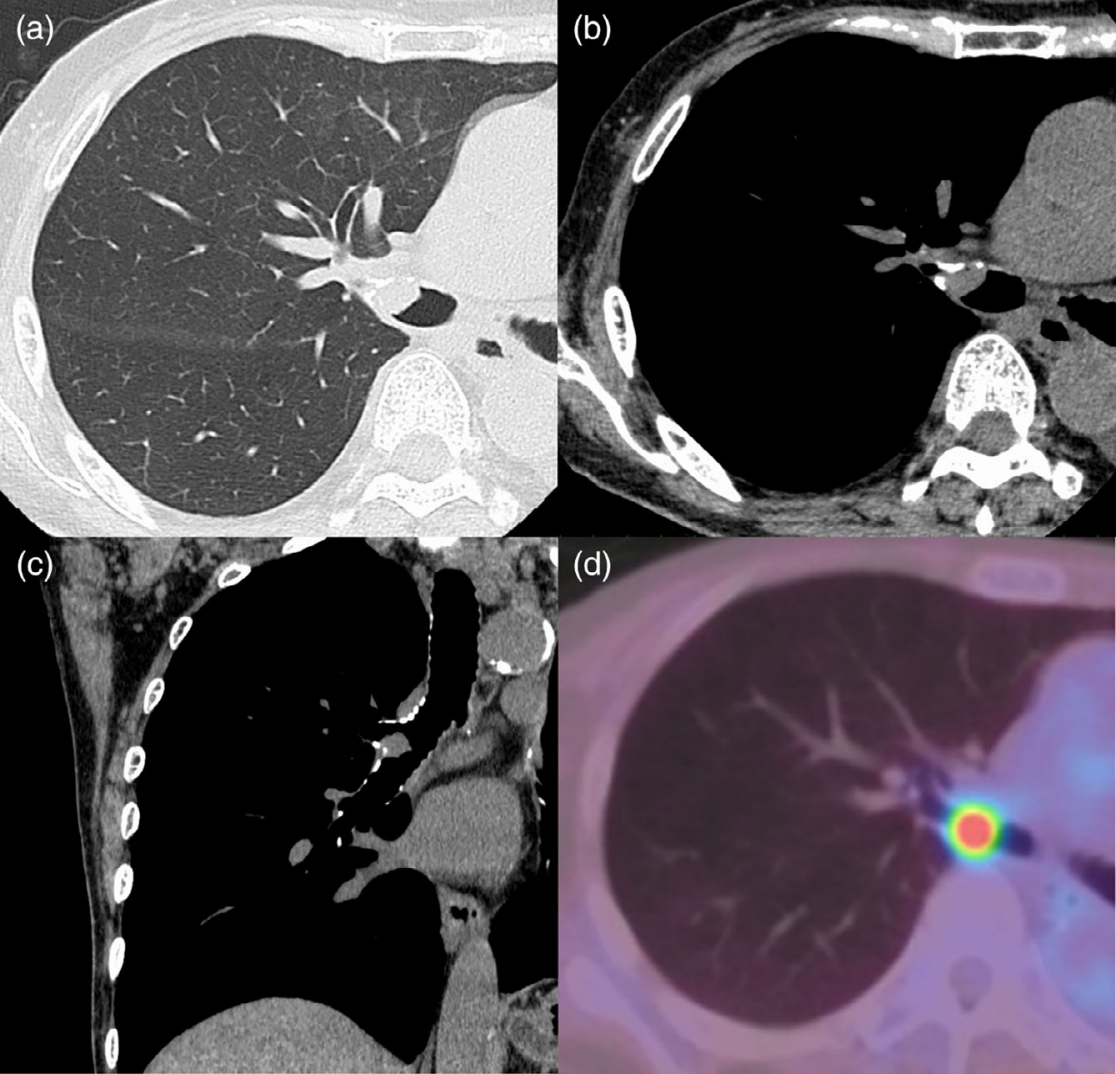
Chest computed tomography (CT) and positron emission tomography‐CT (PET‐CT). Chest CT showed a bronchial tumor with a maximum diameter of 12 mm at the bifurcation of right upper and intermediate bronchi ((a) and (b) axial image, (c) coronal image). The tumor shows high fluorodeoxyglucose (FDG) uptake with maximum standardized uptake value (SUVmax) of 7.2 on PET‐CT (d)
